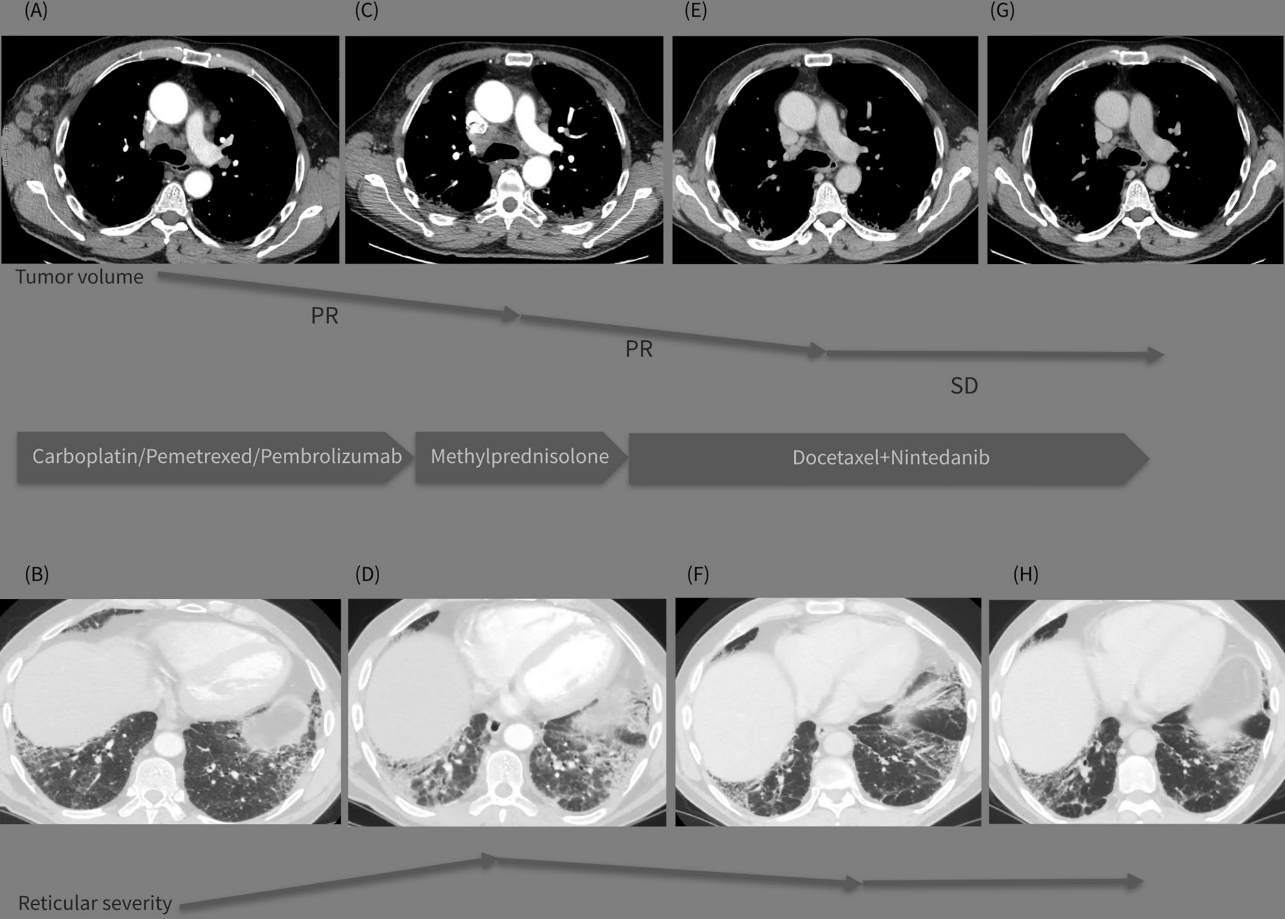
(A–H) Graphic summary of the case. The top line presents sequential CT images on mediastinal window (A, C, E, G) demonstrating the tumor volume regression during the treatment period (Dec 2020-May 2021-Sept 2021-Dec 2021). The bottom line presents images on lung window (B, D, F, H) from the same CT examinations demonstrating the evolution of the interstitial lung abnormalities in the course of the treatment strategy changes (Dec.2020-May 2021-Sept 2021-Dec 2021).
